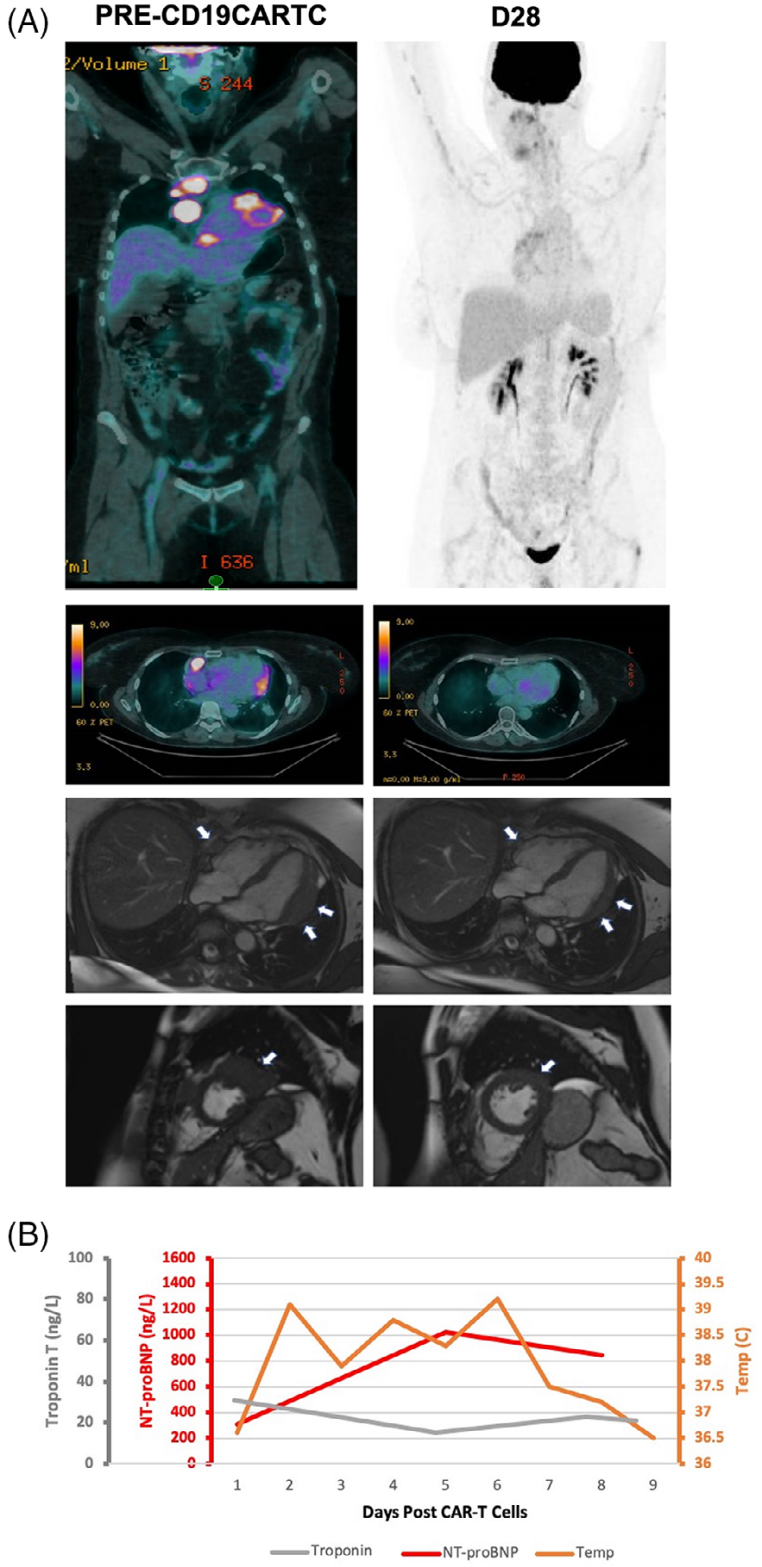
(A) A 46‐year‐old female with relapsed/refractory (r/r) large‐B‐cell‐lymphoma (LBCL) underwent treatment with CD19‐targeting chimeric antigen receptor T‐cell (CD19CAR‐T). FDG‐avid disease within the heart on computed tomography‐positron emission tomography (CT‐PET) at baseline, corresponding to multifocal myocardial and pericardial lymphomatous infiltration on cardiac magnetic resonance imaging (CMR) including an intramyocardial lateral left ventricle (LV) mass measuring 24 × 31 mm. CT‐PET at day 28 post‐CD19CAR‐T demonstrated a Deauville 4 response and regression of the intramyocardial LV wall mass without evidence of myocardial edema on CMR. (B) Fevers and grade 1 CRS were associated with an elevated NT‐proBNP; TnT levels did not change.
